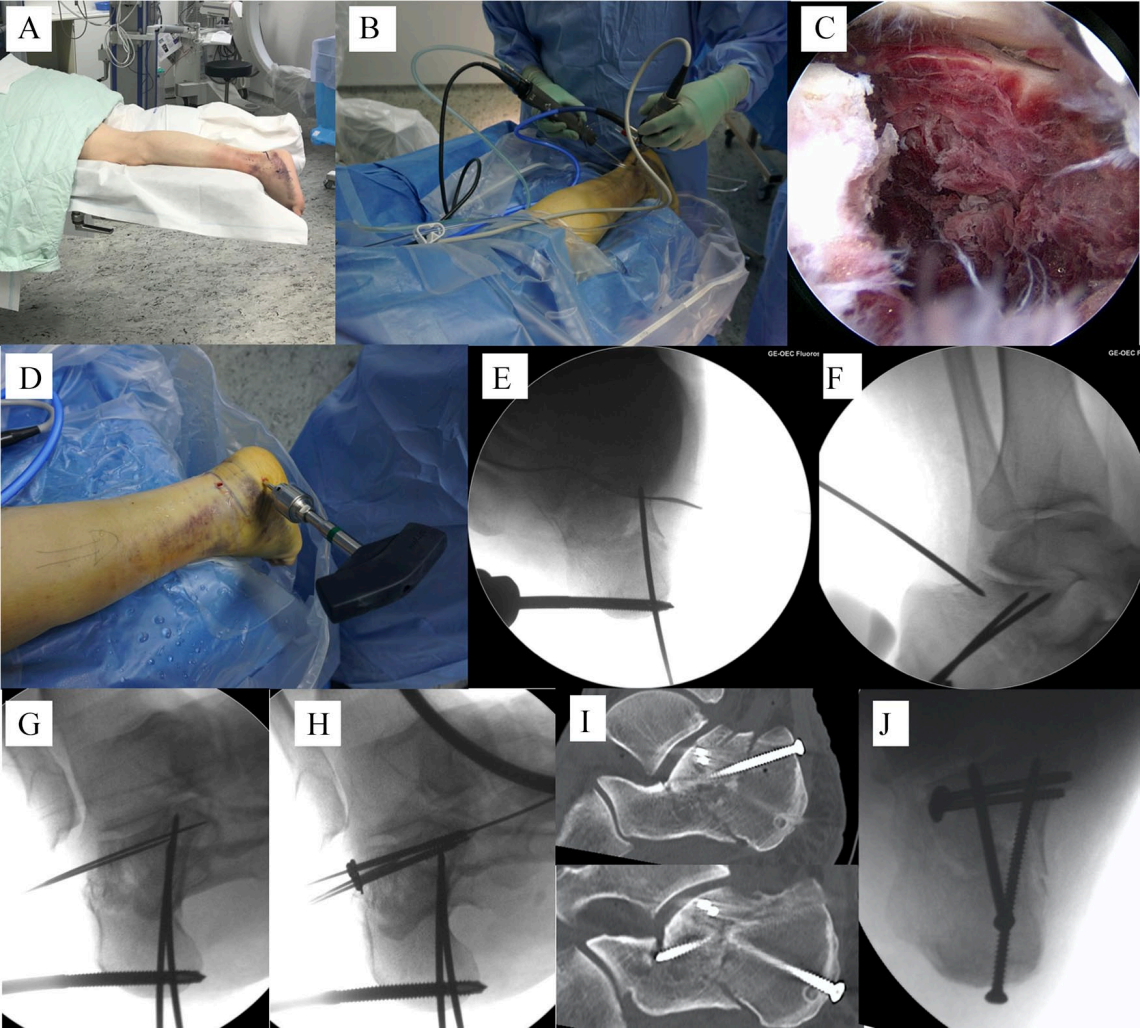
Operative technique. (a) Positioning of the patient. (b) Posterior ankle arthroscopy. (c) Entering the fracture and the subtalar joint. (d) Placing a Schantz screw in the tuber fragment. (e) Reduction of the tuber fragment and temporary fixation. (f) Reduction of the posterior facet fragment(s). (g) Temporary stabilization of the joint fragment(s). (h) Screw fixation of the joint fragments. (i) postoperative sagittal computed tomography scans. (j) Postoperative axial fluoroscopy.
